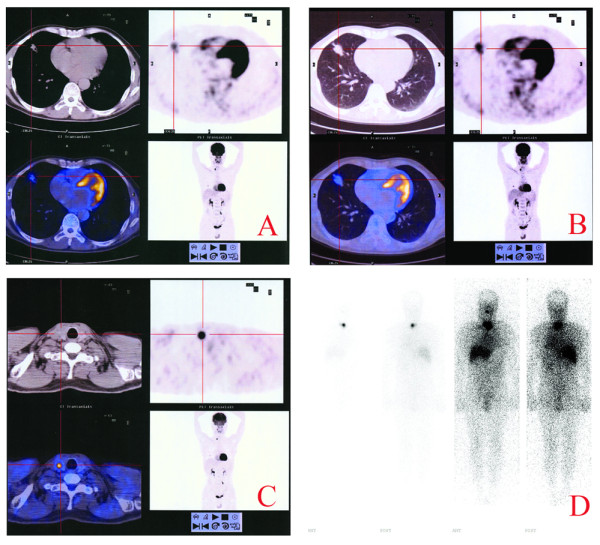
Whole-body FDG PET/CT and radioiodine scan. A hypermetabolic focus in the mid lobe of lower lobe of the right lung with increased FDG uptake in SUV max 3.69 (A) and delayed SUV 5.17 (B). PET/CT-detected round hypermetabolic focus in the right lobe of the thyroid with abnormal FDG uptake in SUVmax 19.97(C). Postoperative therapeutic dose ^131^I whole body imaging showed mild accumulation of radioiodine (T/NT 3.64) in the residuary thyroid, while no abnormal ^131^I uptake in the other side (D).
